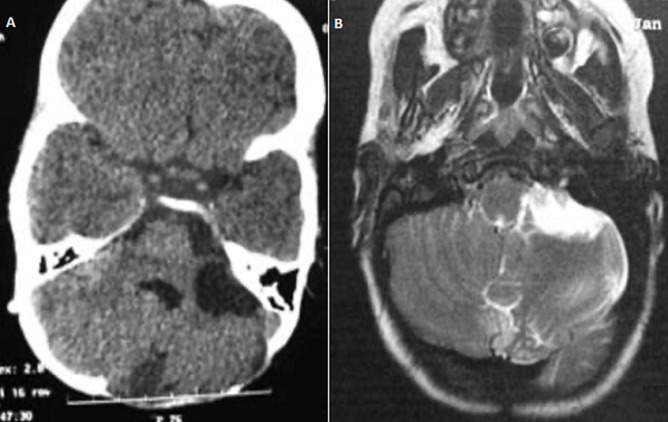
A) cerebral CT scan; B) cerebral MRI show a collapse of the arachnoid cyst from the cerebellopontine angle and total excision of the vermian dermoid cyst
